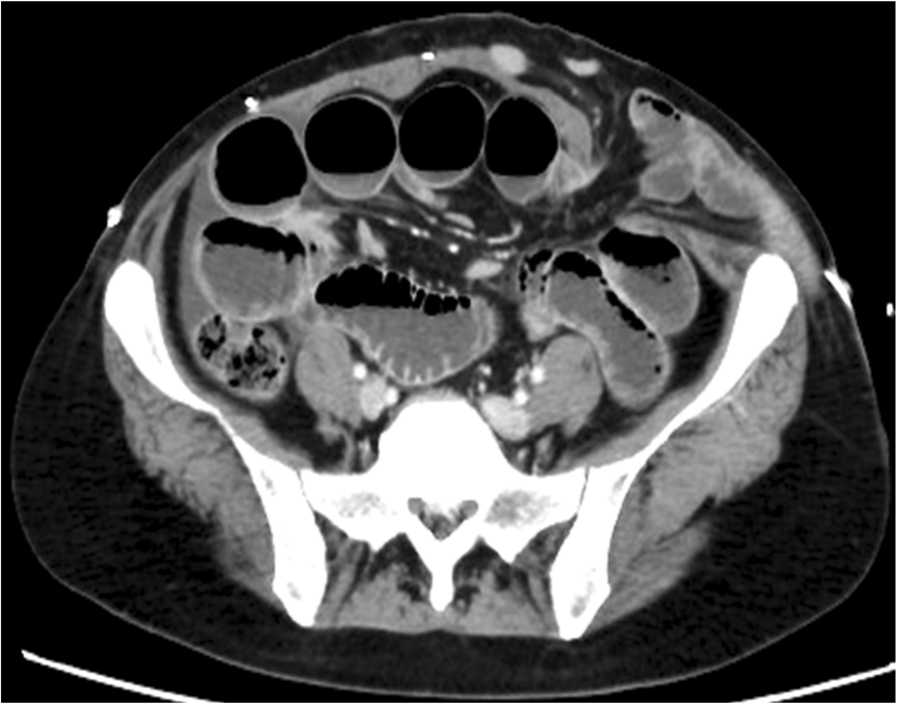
Abdominal contrast-enhanced computed tomography showing bowel herniation into the subcutaneous tissue
